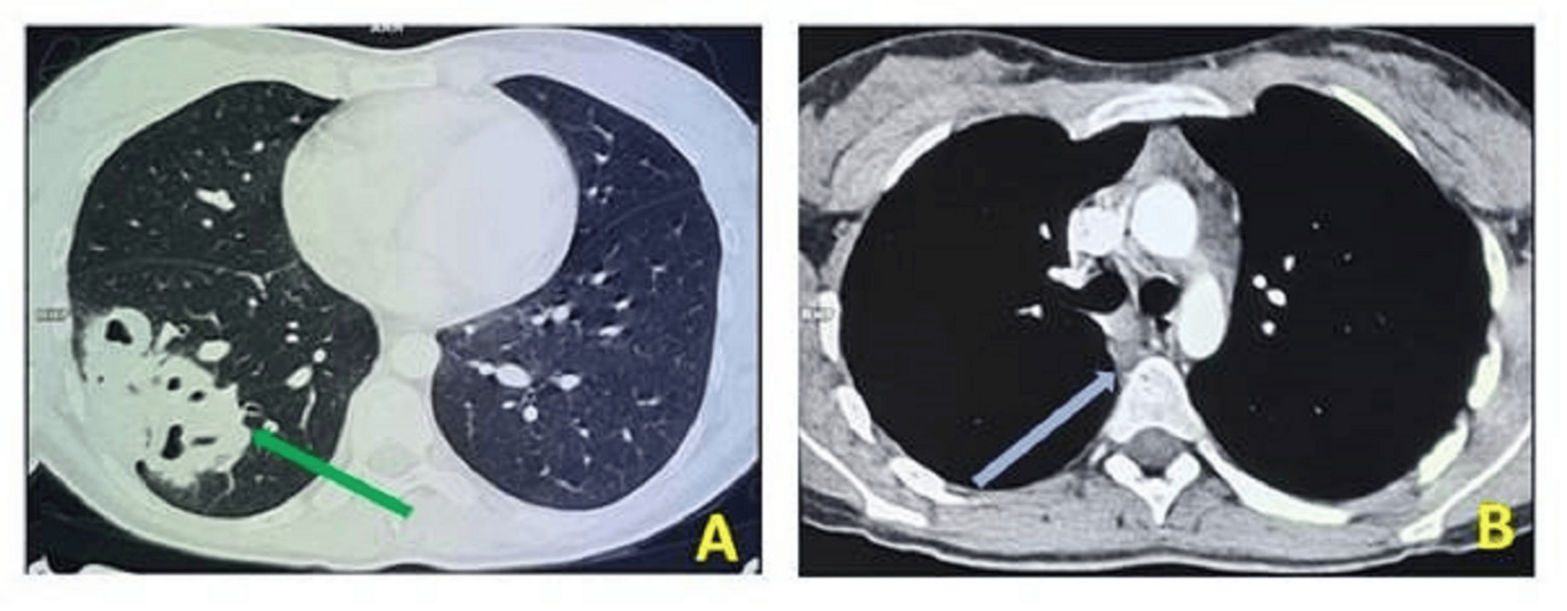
Thoracic CT image showing a heterogeneous mass with cavitation in the right lower lobe (green arrow), accompanied by Baréty's lodge adenopathies and subcarinal adenopathies (blue arrow).
